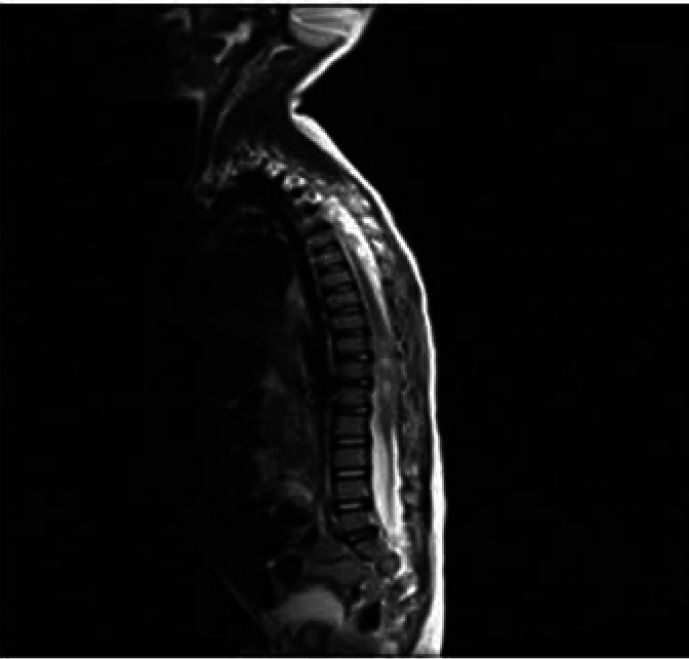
lumbar magnetic resonance imaging (MRI) without contrast that shows a dumbbell shape mass, the hyper signal in T2-weighted image and low signal in T1- weighted image, extramedullary, intramural with mass effect on the cord
